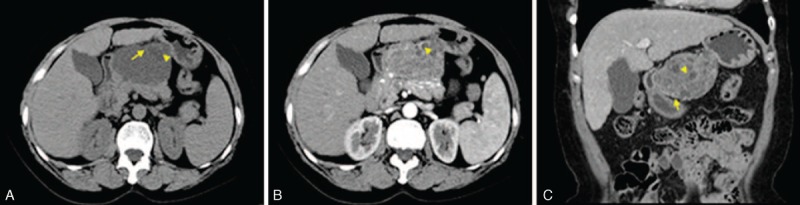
Abdominal CT demonstrating a heterogenous mass (arrow) at the neck and body of the pancreas, adjacent to the descending portion of duodenum and the lesser curvature of the gastric body. The cystic part has internal division change (arrowhead) (A). The mass, cystic wall (arrowhead), and internal division (arrow) could be seen intensified on contrast-enhanced CT images compared with those on precontrast images (B and C). CT = computed tomography.
